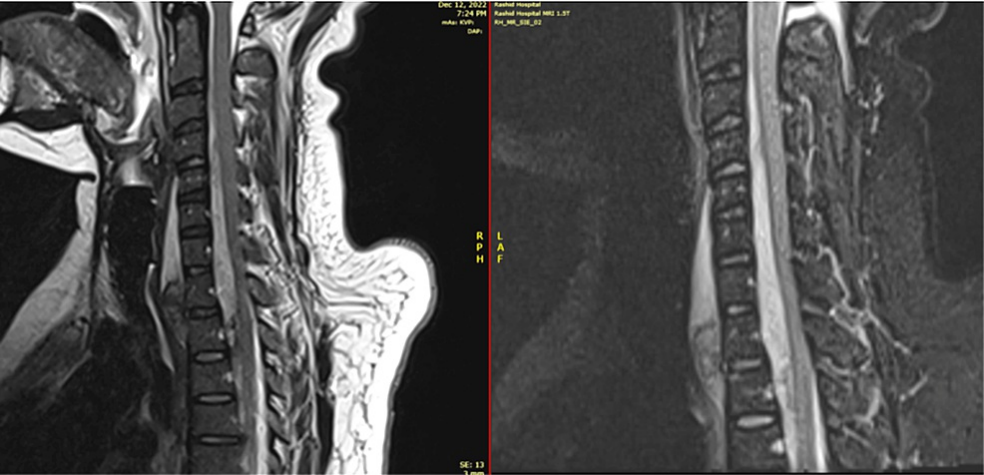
MRI sagittal T2 and STIR images reveal large prevertebral and intraspinal epidural hematoma (C5-T2) with spinal cord compression. STIR: Short Tau Inversion Recovery
